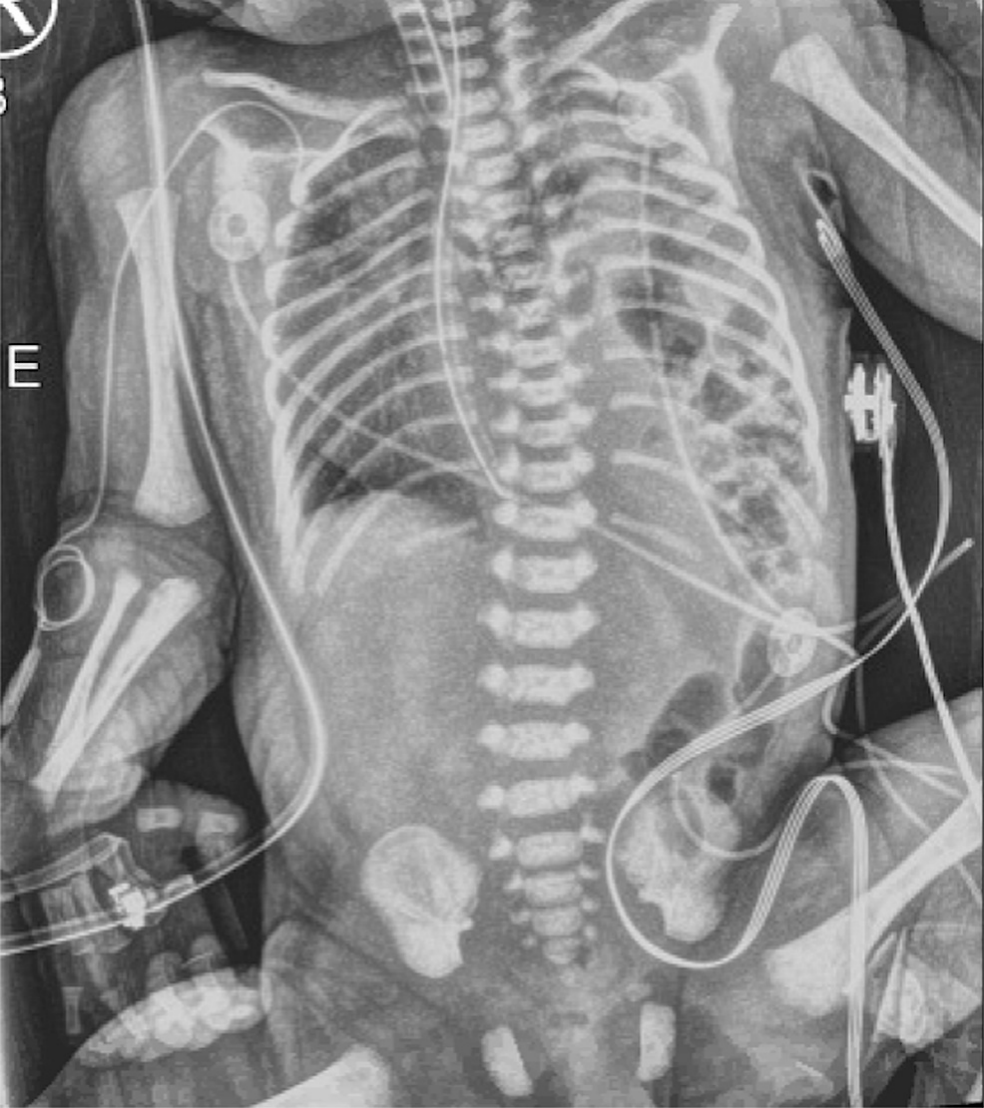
Preoperative anteroposterior radiograph of infant demonstrating a left-sided congenital diaphragmatic hernia with resultant dextrocardia
